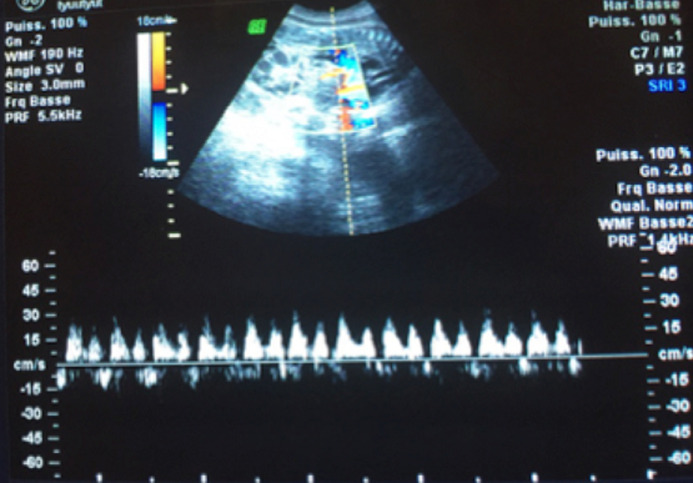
doppler du ductus venosus objectivant une onde à négative
